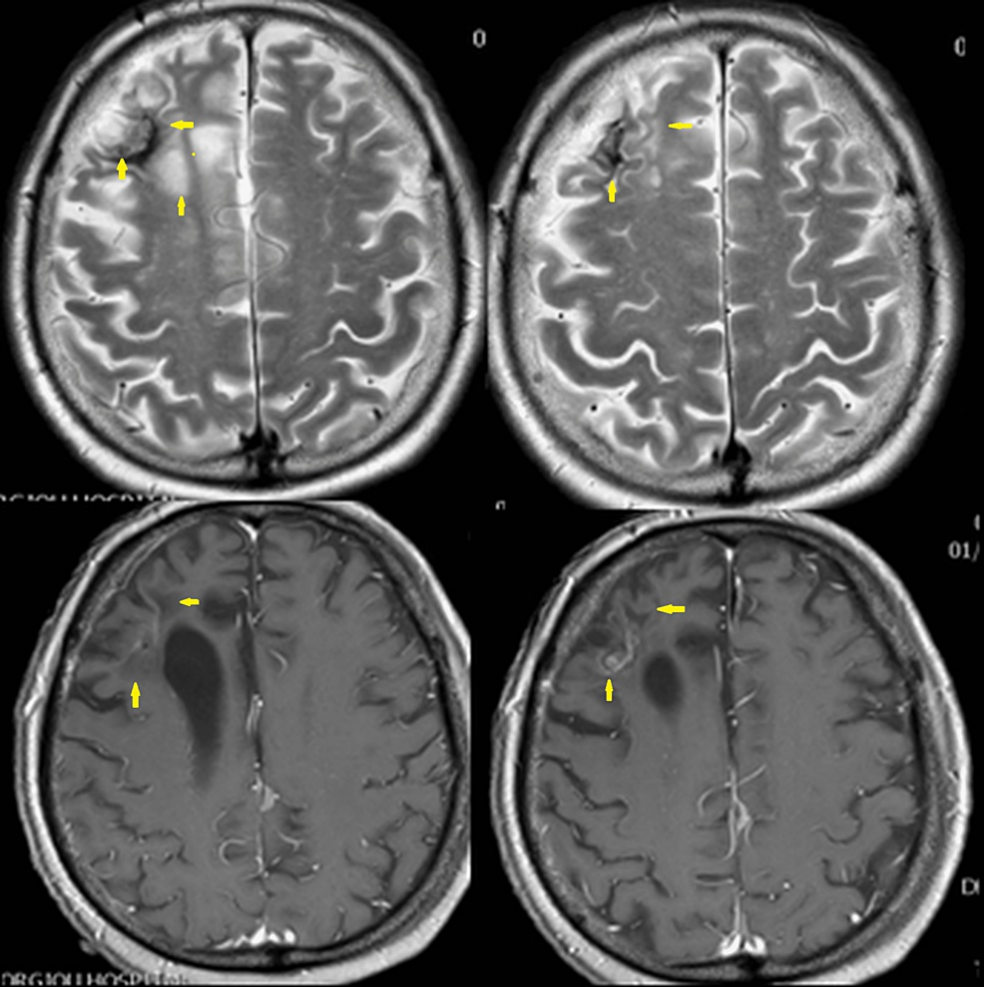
Brain magnetic resonance imaging on January 10, 2018. Gliotic and calcified right frontal lobe lesions. No other lesions are revealed.
